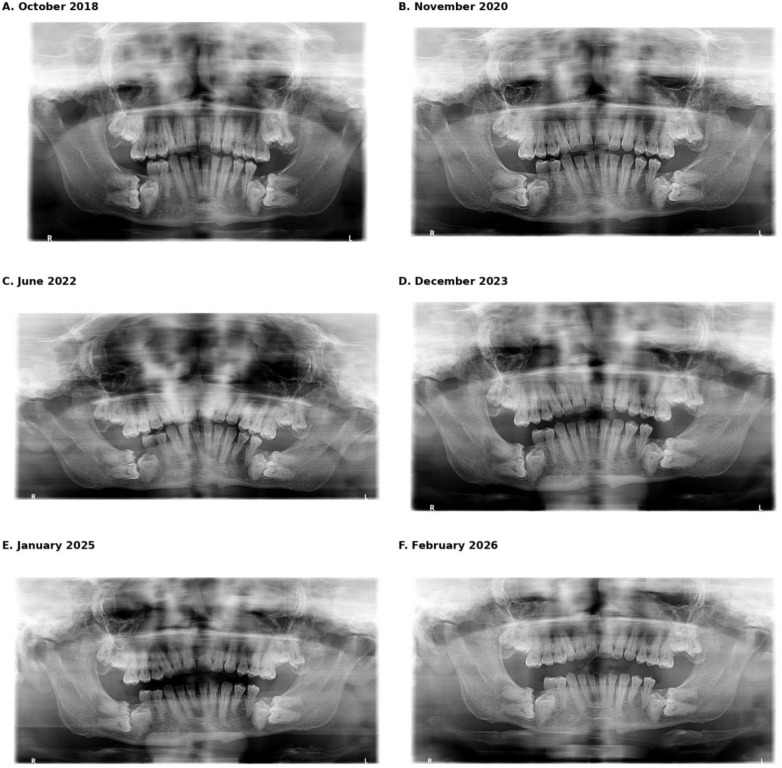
Serial panoramic radiographs obtained between 2018 and 2026 showing progressive bilateral temporomandibular joint remodeling in an adult patient with genetically confirmed NAO syndrome. (A) October 2018: bilateral flattening of the superior condylar contours and articular eminences with shallow glenoid fossae; cortical outlines remain continuous. (B) November 2020: persistent condylar flattening with mild lateral osteophytic change, more conspicuous on one condyle, and greater flattening of the articular eminences. (C) June 2022: further bilateral flattening of the condylar surfaces with increased apparent shallowing of the glenoid fossae. (D) December 2023: no major interval change relative to 2022. (E) January 2025 and (F) February 2026: advanced remodeling with irregular but continuous cortical outlines of the condyles and marked flattening of the articular eminences with loss of normal posterior slope. Interpretation is limited by inherent magnification, distortion, and superimposition of panoramic imaging and by incomplete visualization of the left TMJ in several panels. Only one unlabelled set of radiographs (A–F) is provided.
